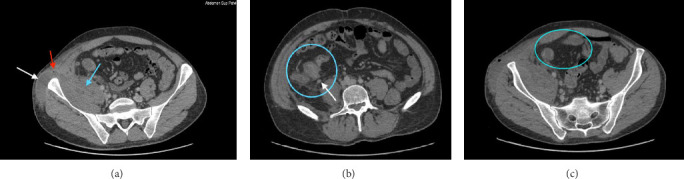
(a) Contrast-enhanced CT of the abdomen and pelvis showing signs suspicious of iliopsoas abscess secondary to Crohn's disease: white arrowhead–abscess extension. Red arrowhead—fistulous tract connecting the external collection of pus with the abscess at the terminal ileum (blue arrowhead). Only intravenous contrast medium had been used, and no oral contrast was given. (b) Contrast-enhanced CT of the abdomen and pelvis showing signs suspicious of iliopsoas abscess secondary to Crohn's disease: blue circle—thickening of terminal ileum. White arrowhead—enlarged lymph node. Only intravenous contrast medium had been used, and no oral contrast was given. (c) Contrast-enhanced CT of the abdomen and pelvis showing signs suspicious of iliopsoas abscess secondary to Crohn's disease: blue circle—thickening of terminal ileum. Only intravenous contrast medium had been used, and no oral contrast was given.
